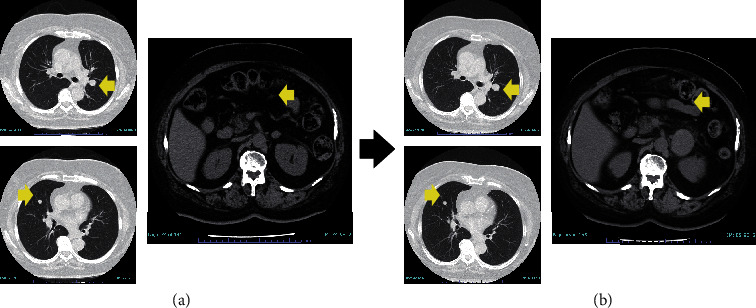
Computed tomography of lung tumors and a left adrenal tumor (arrows). (a) At the time when the left adrenal tumor was initially identified. (b) Six months after the initial diagnosis of the left adrenal tumor.
